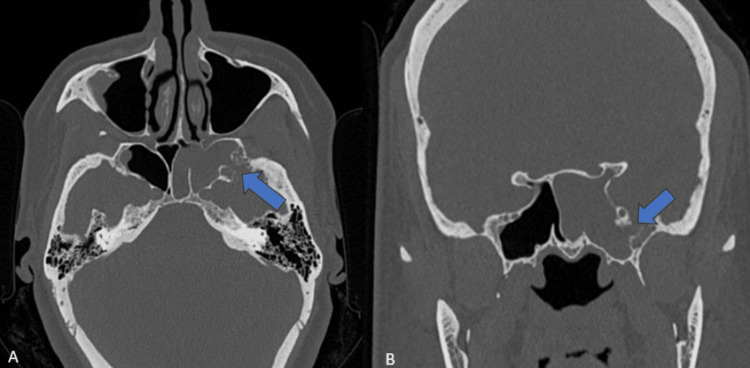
Axial and coronal CT sections at the level of the sphenoid sinus A: axial CT section; B: coronal CT section shows a focal defect (blue arrow) in the left lateral recess of the sphenoid sinus. There is complete opacification of the left side of the sphenoid sinus with fluid.
